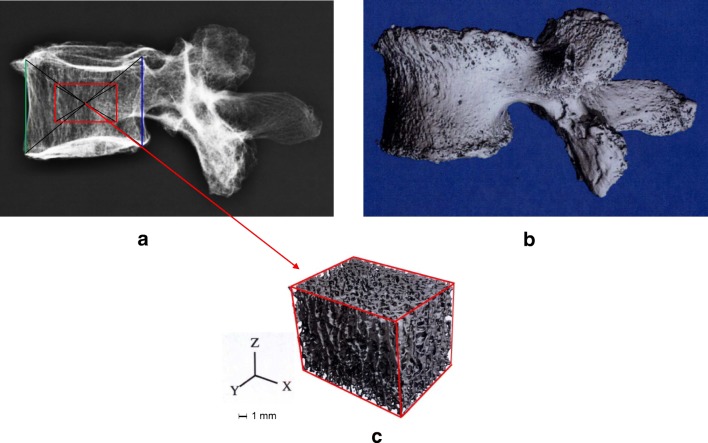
Panel showing ex vivo images of one and the same vertebra (lumbar vertebra 4 of an 87-year-old woman) taken with conventional radiograph (a), and high-resolution computed tomography (HR-pQCT; b). c showing the three-dimensional region of interest used for the assessment of histomorphometric parameters (e.g., trabecular bone volume)
